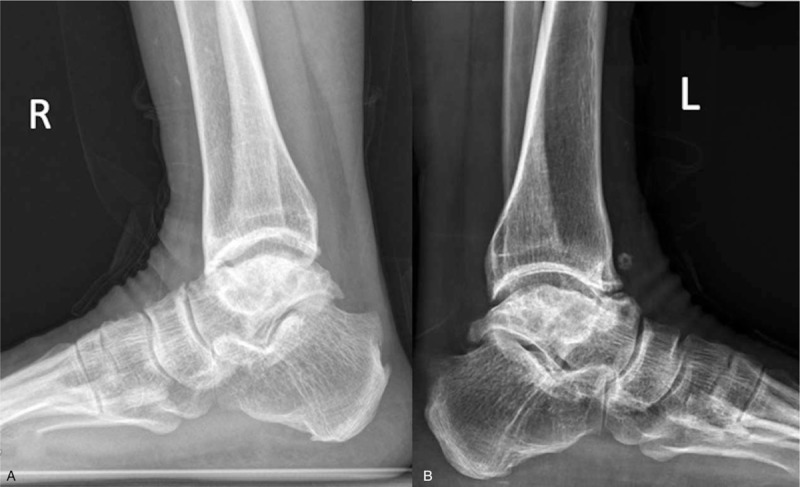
The radiographs demonstrated partial talar avascular necrosis (AVN) with collapse and sclerotic changes of the talar body on both sides. Characteristic calcaneal shortening deformity was demonstrated on both ankle radiographs (A–B). Osteophytes and loose bodies were demonstrated in the left ankle joint (B).
